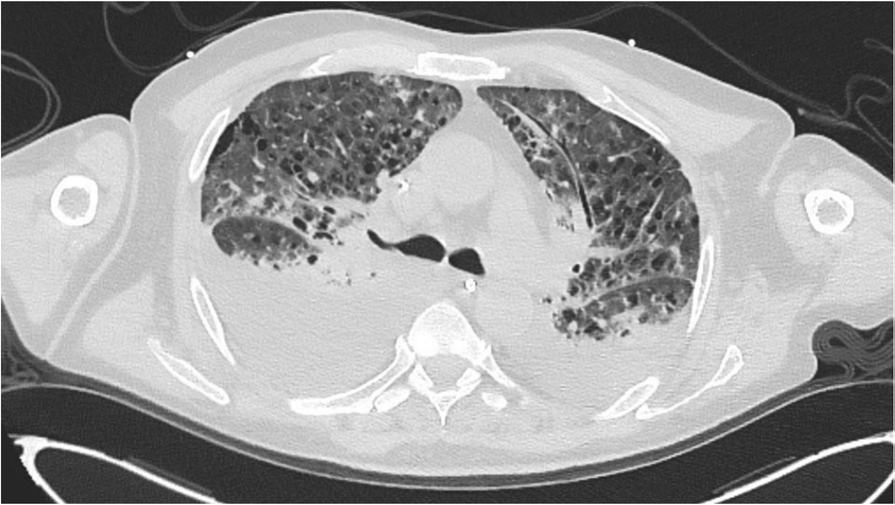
CT scan at hospital admission. Chest CT scan shows extensive bilateral pulmonary infiltrates with large pulmonary effusions and mediastinal lymphadenopathy
